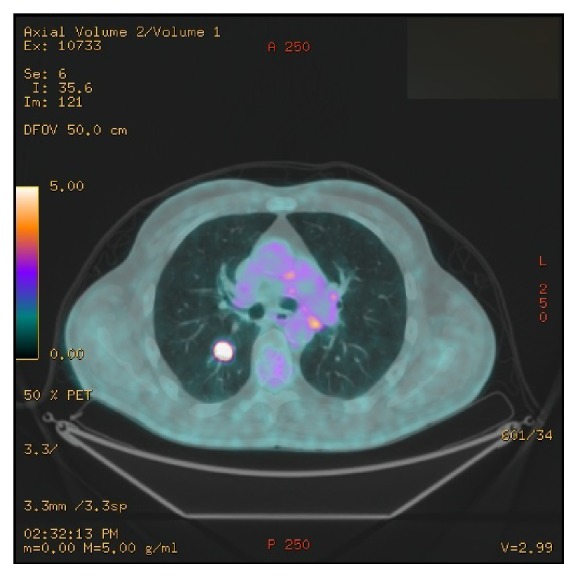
18F-FDG PET-CT scan showing a highly suspicious lesion in the right lung.
